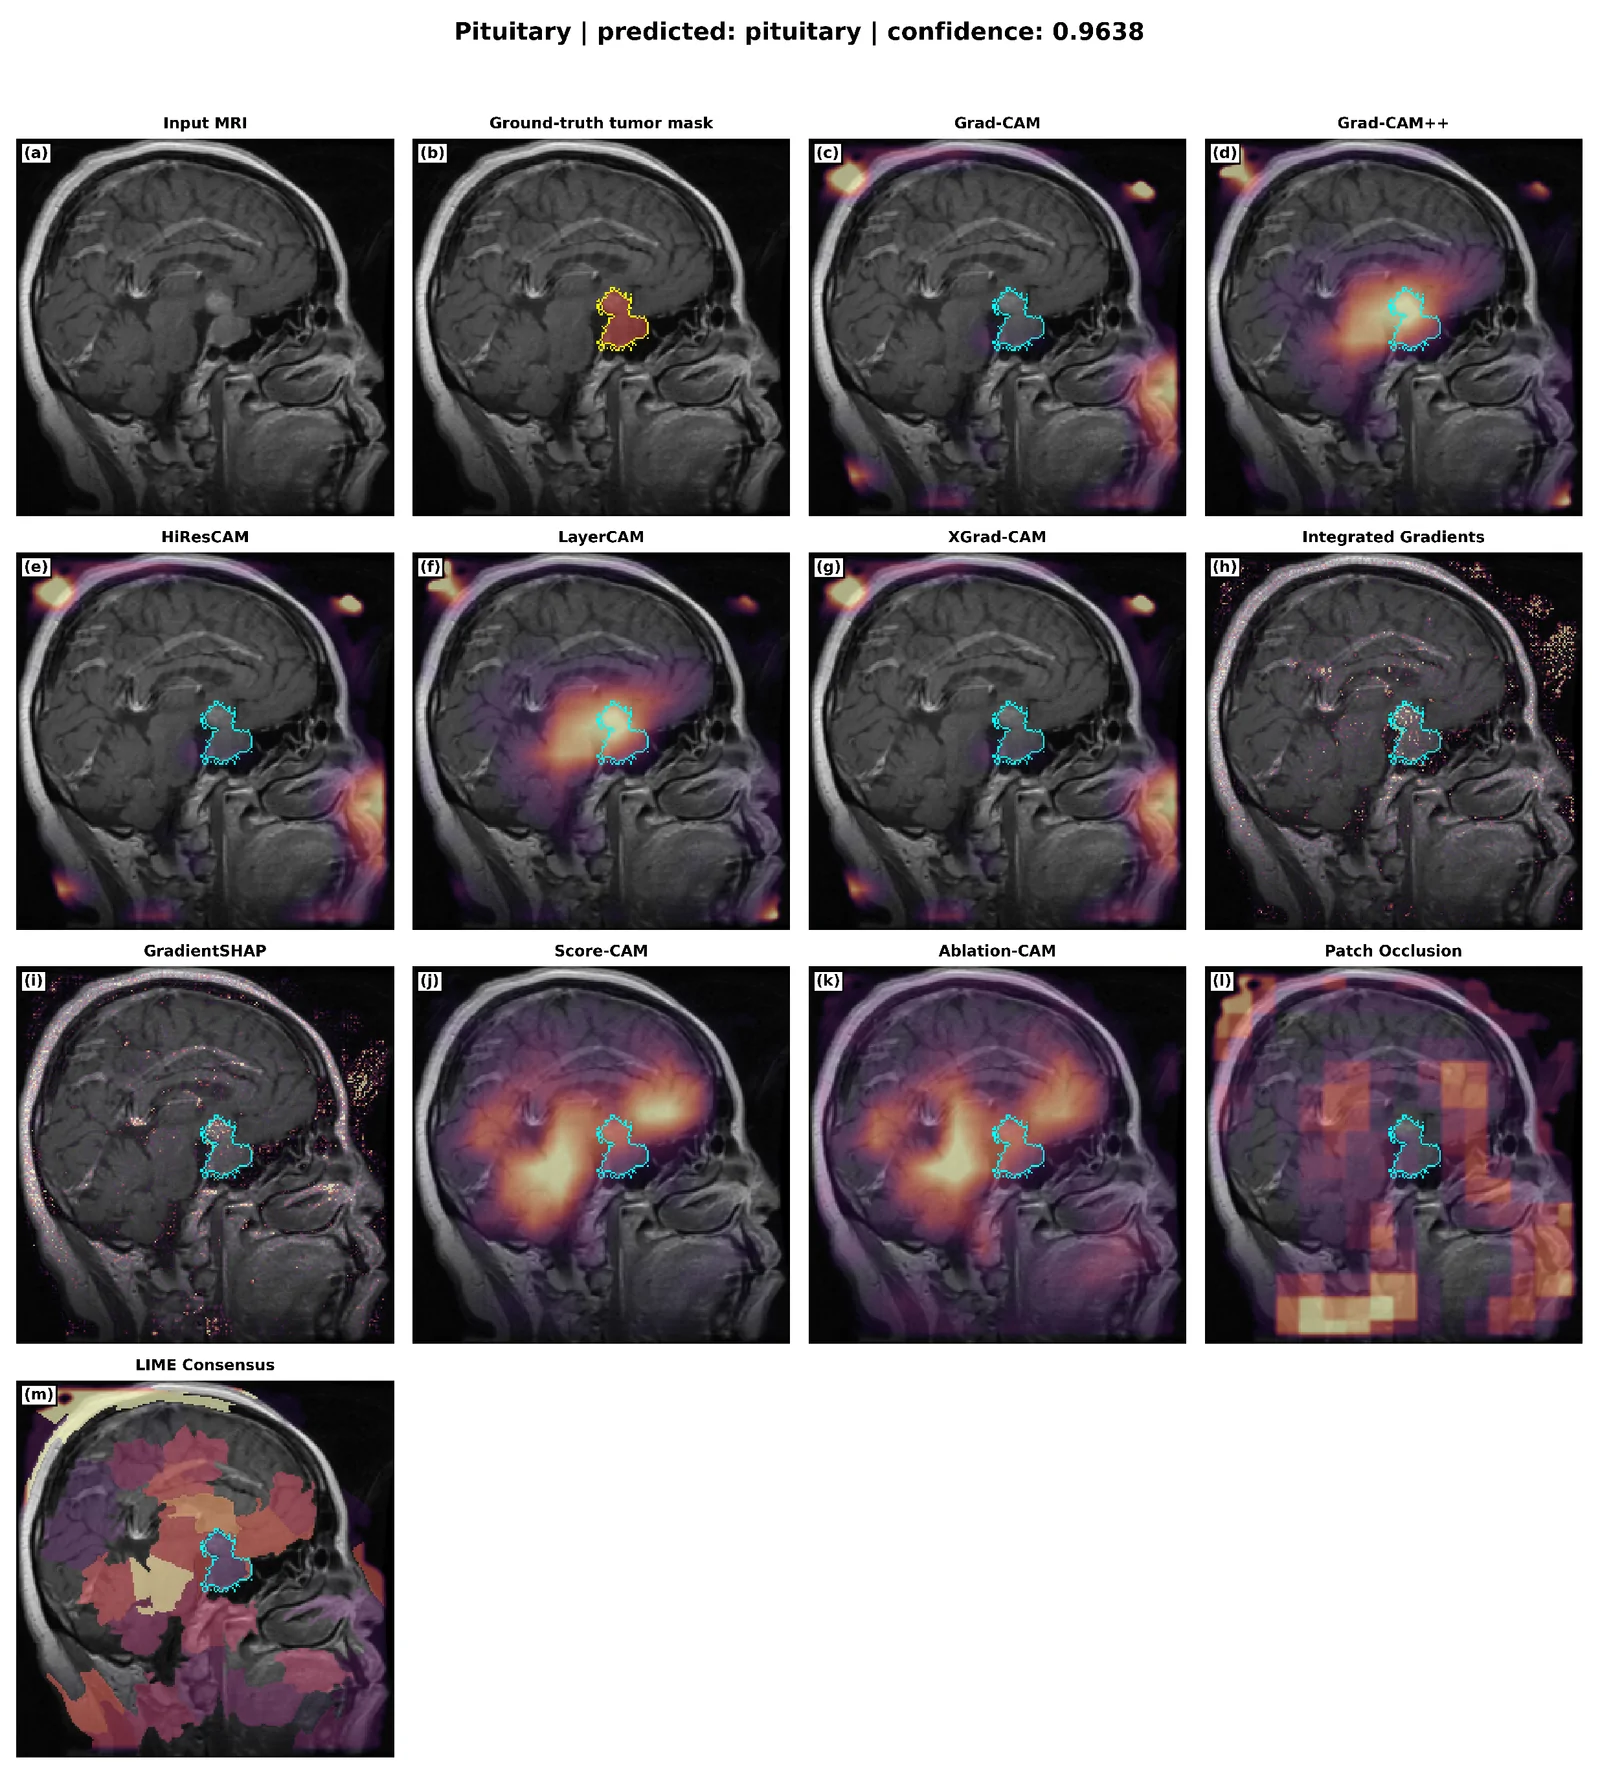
Visualization of all evaluated XAI methods for a pituitary tumor MRI image.
